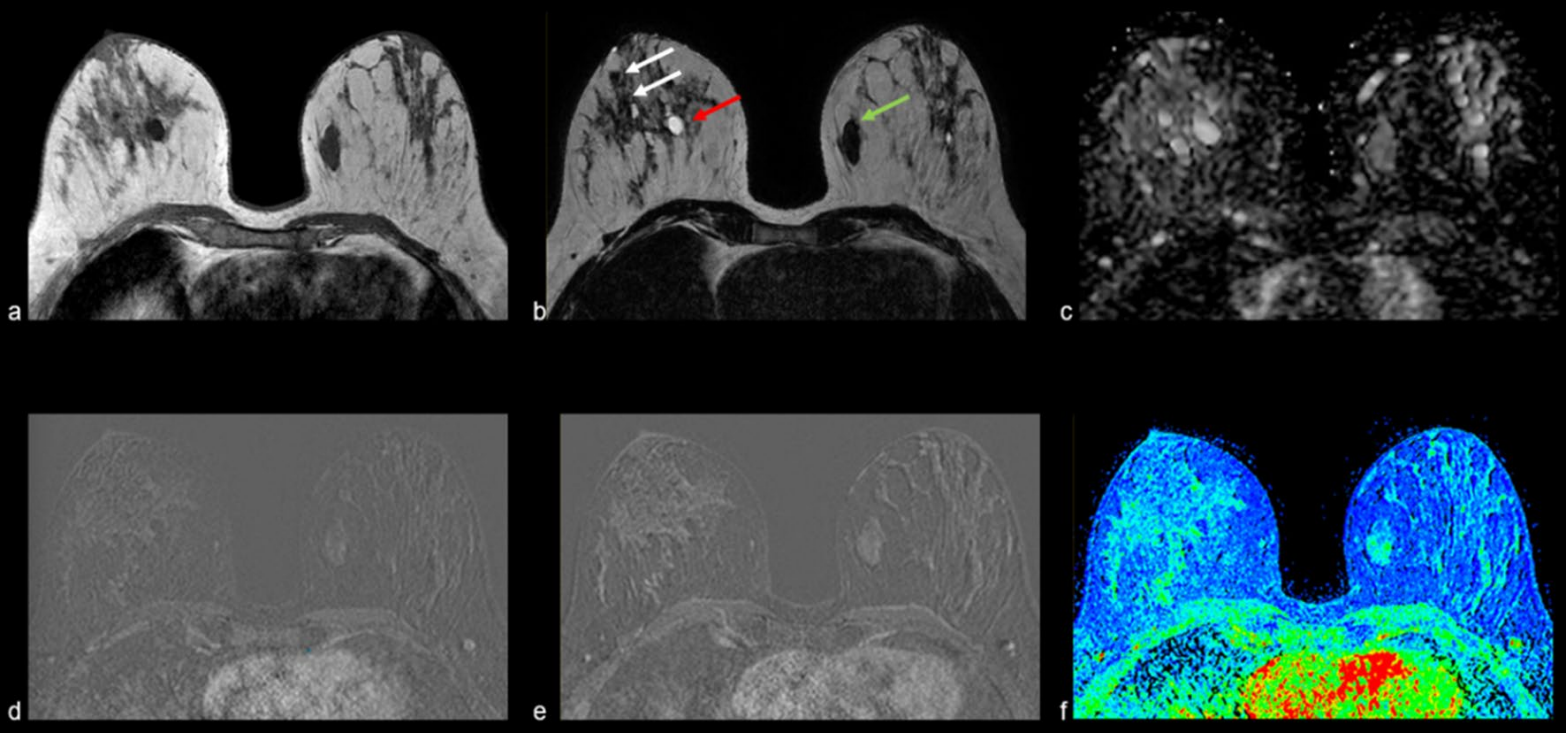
Example of MRI findings of breast lesions categorized as BIRADS 0 and BIRADS 3 by previous examinations. A 43-year-old patient with multiple bilateral findings, most of them simple cysts (white arrows). At the left breast (green arrow), a solid lobulated lesion, hypointense on T1W (a) and T2W (b) sequences, which shows an ADC (c) of 1.43 × 10^−3^mm^2^/s, with the contrast medium it shows an homogeneous wash-in pattern (f), with a slight progressive uptake from the initial (d) to delayed (e) postcontrast subtractions, corresponding to a benign lesion with characteristics of fibroadenoma. At the right breast (red arrow), a nodule, hypointense on T1W (a) and hyperintense on T2W (b), which shows no restricted diffusivity and no contrast enhancement. Both lesions were categorized as BIRADS 2
